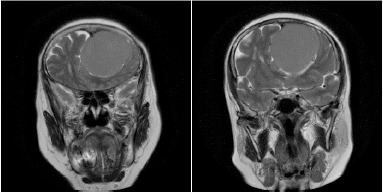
The brain MRI showed a frontal lobe isointense mass
